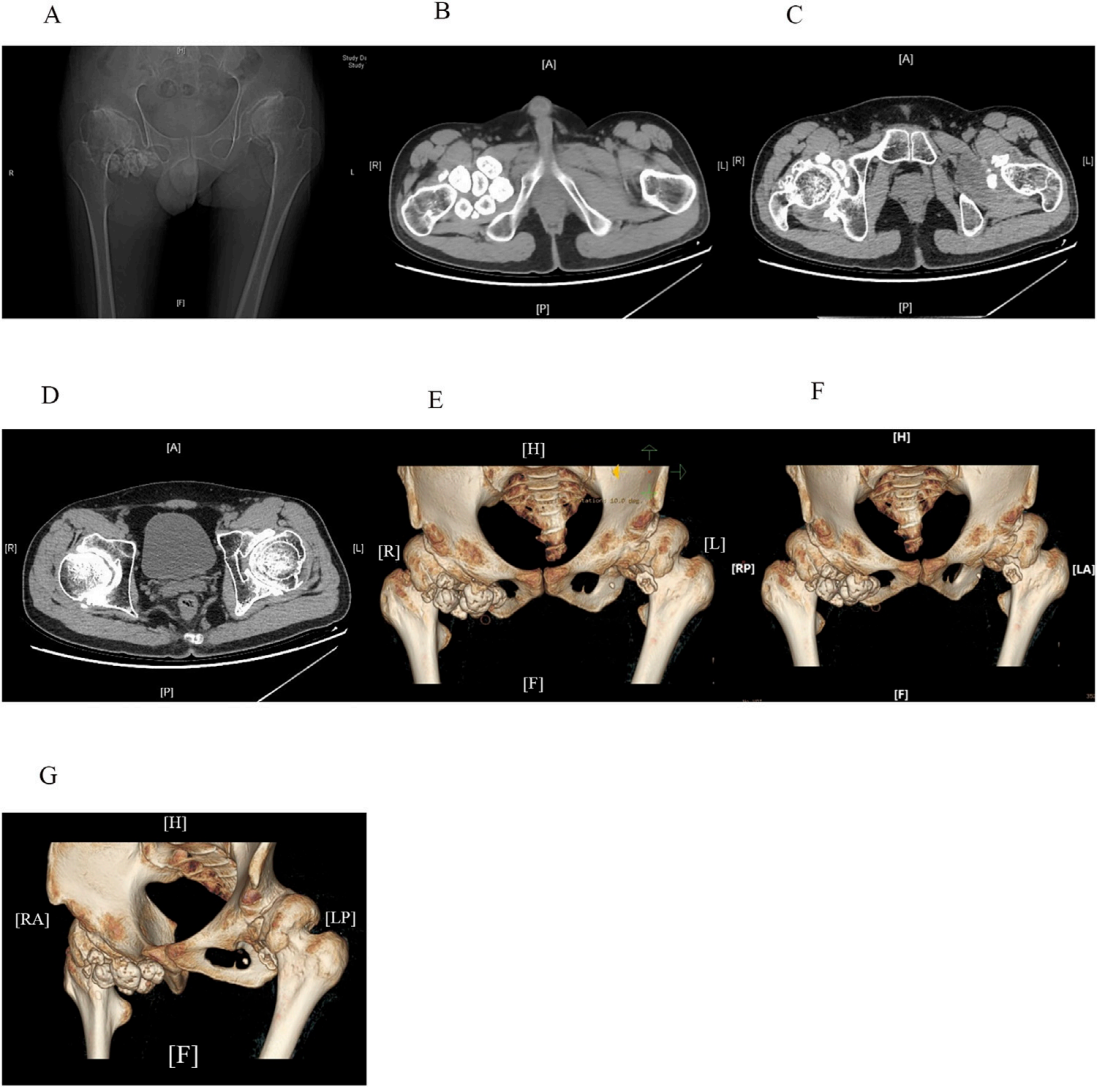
CT scans (A–D) and three-dimensional reconstruction images (E–G) of the hip joints reveal shallow acetabula with increased acetabular indices and bone hyperplasia along the joint margins bilaterally. The femoral heads appear hypertrophic and flattened, with anterolateral displacement and thick, short femoral necks. The neck-stem angle of the femur is increased, and the hip joint spaces are nonuniformly narrowed. Articular surface sclerosis and subarticular cystic changes are present on both sides. Enlargement of the bilateral hip joint capsules is evident, with multiple pomegranate seed-like high-density foci, particularly on the right side. Soft tissue swelling can also be observed around the joint.
